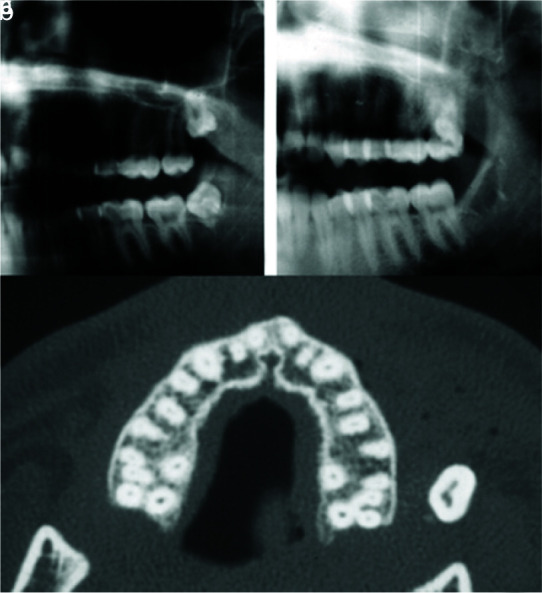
a: pre-operative panoramic x-ray showing the maxil-lary left impacted molar in place before the surgery. b: post-operative panoramic x-ray showing
